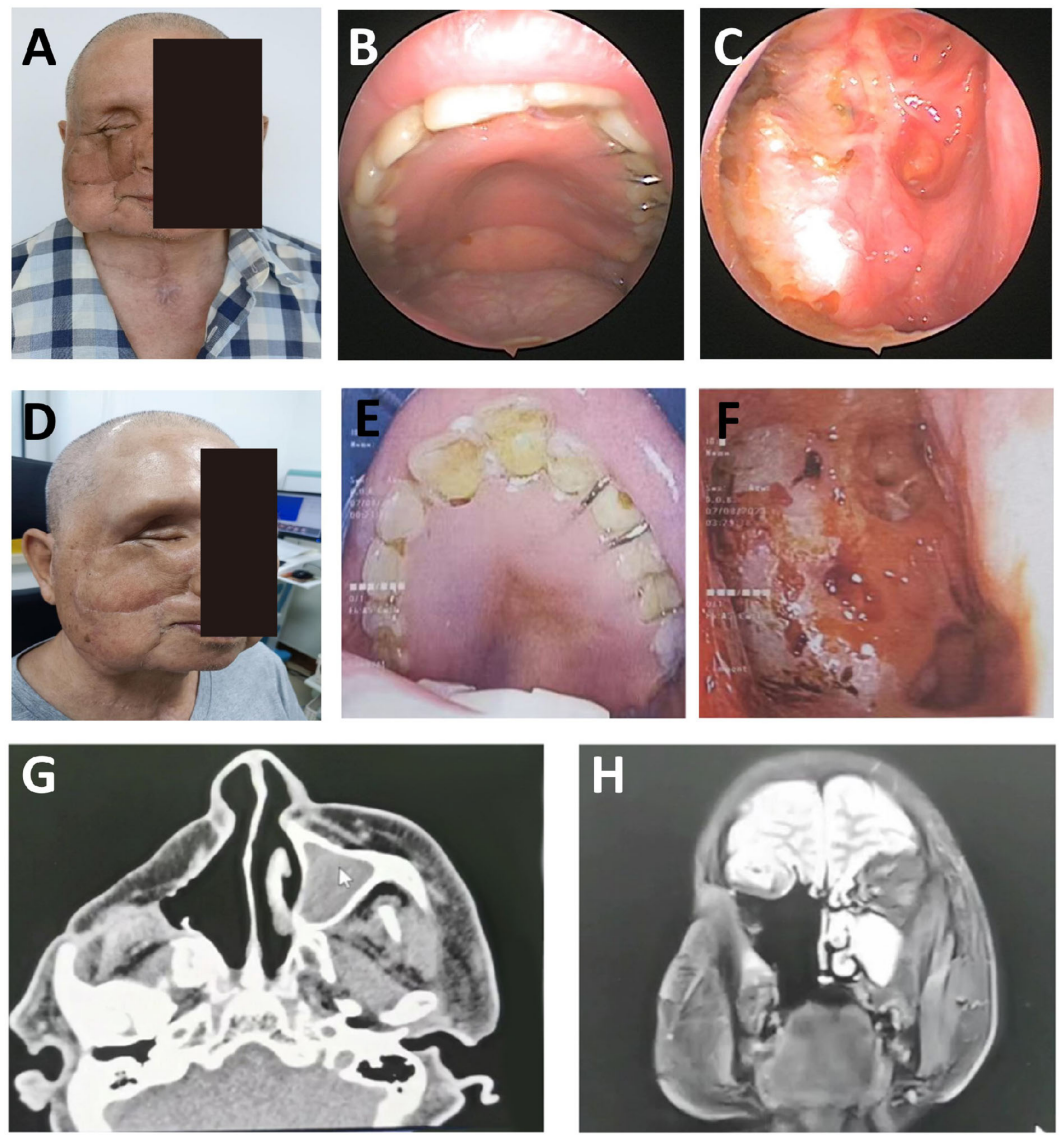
Dynamic evaluation of facial reconstruction outcomes at 6 and 18 months postoperatively in Case 1. (A–C) 6 months postoperative: Frontal, lateral, and oblique views showing complete integration of the SMAPF with recipient tissues (integration rate 100%). Donor site scar width was measured at 1.8 ± 0.3 mm using calipers. Infrared thermography revealed flap surface temperature variation within ≤1.5 °C. (D–H) 18 months postoperative: Multiview assessments demonstrate uniform flap color without contraction or distortion (3D facial scan asymmetry deviation <4%). Paranasal sinus CT images. (G, H) confirm stable bony structure with no soft tissue overgrowth or signs of tumor recurrence.
